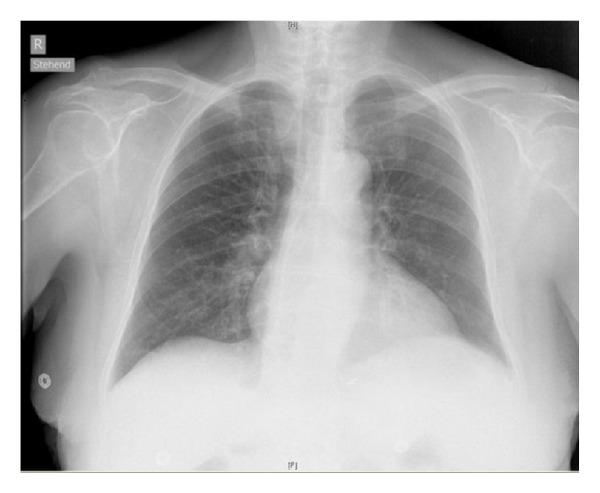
Chest X-ray anteroposterior at admission (sitting).
